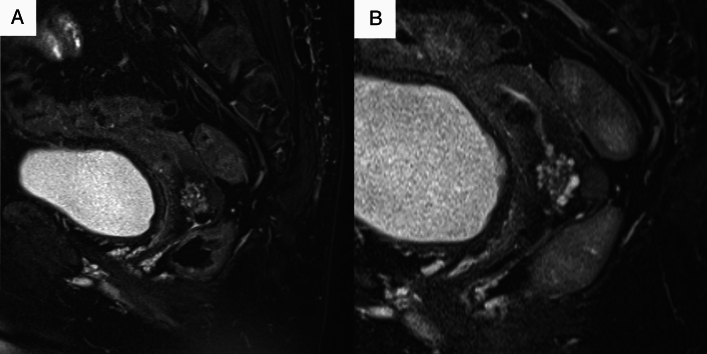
A Sagittal T2-weighted (T2WI) image of a 64-year-old woman (postmenopausal) with atypical lobular endocervical glandular hyperplasia showing a cystic aggregate lesion in the upper part of the cervix with numerous small cysts. This lesion was classified as the “microcystic pattern.” B Follow-up of this patient at the age of 66 years (postmenopausal) shows that the lesion did not change in size, and the microcystic pattern persisted
